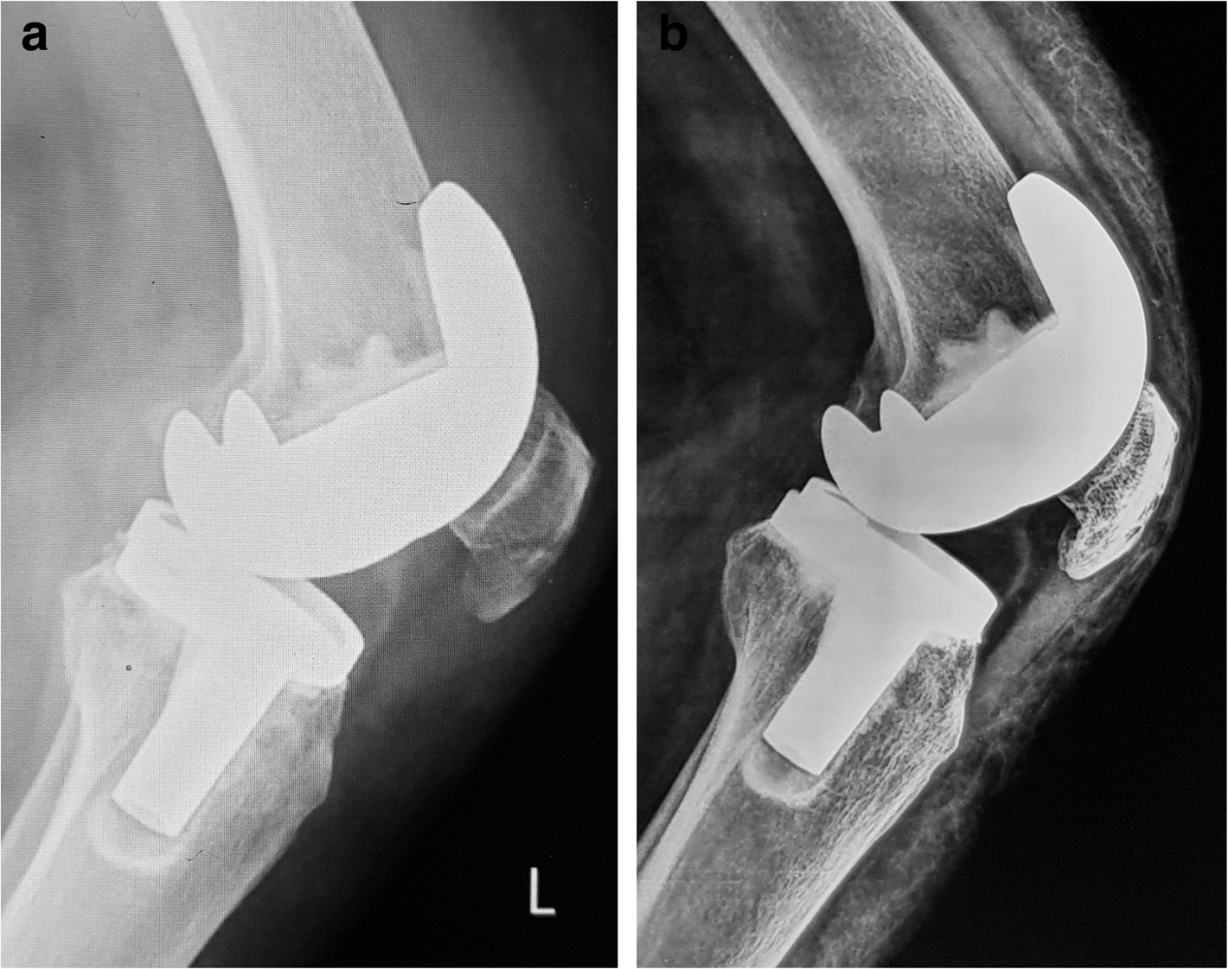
A 64-year-old female who suffered a PPPF in her left knee (case 4 in Table 1). a Preoperative lateral X-ray shows a posterior stabilized system (Depuy) and minimally displaced fracture. b The fracture is managed conservatively with good union one year later
